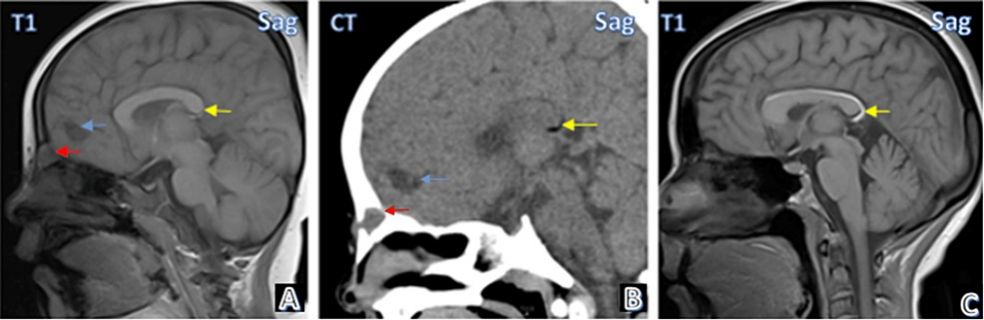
Case gallery Case 3: Frontal meningoencephalocele (red arrow), curvilinear lipoma along posterior aspect of corpus callosum (yellow arrow), focal frontal cystic encephalomalacia (blue arrow) seen on MRI (A) and CT (B). Case 4: Lipoma along the entire extent of the corpus callosum(C). (Yellow arrows depict lipomas in all images)
